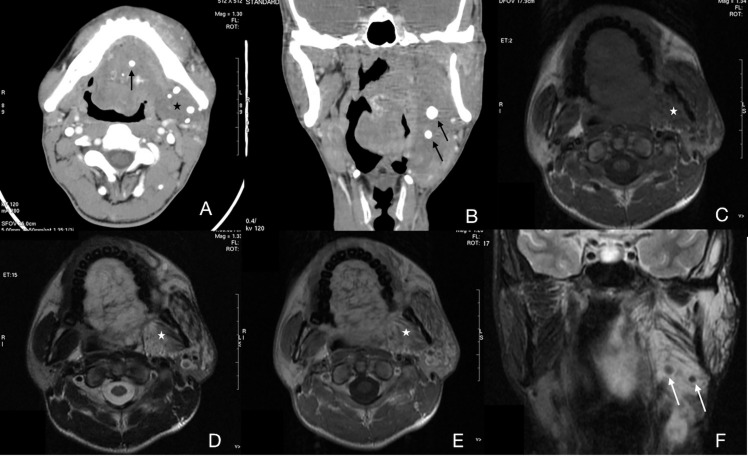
Case 13 (Supplementary Table 1), cavernous IMHs. (A) Axial CT image showing multiple phleboliths (arrow) in a diffuse, hypo attenuated lesion (star). Oral floor and left masticatory muscles were infiltrated. (B) Coronal CT image showing multiple phleboliths (arrow). (C) Axial T1WI showing a diffuse, intermediate signal-intensity lesion with a vague border (star). (D) Axial T2WI showing a lesion with slightly high signal intensity and a blurred border. (E) Axial enhanced T1WI showing no enhancement of the lesion (star). (F) Coronal enhanced fat-suppressed T1WI showing multiple round, low signal-intensity structures which indicated phleboliths (arrow).
